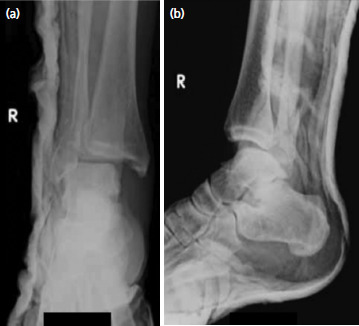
Patient 1 – (a) Antero-posterior and (b) lateral radiographs on arrival showing a short oblique lateral malleolar fracture and postero-lateral subluxation of the talus with entrapment of the proximal fibula behind the tibia.
